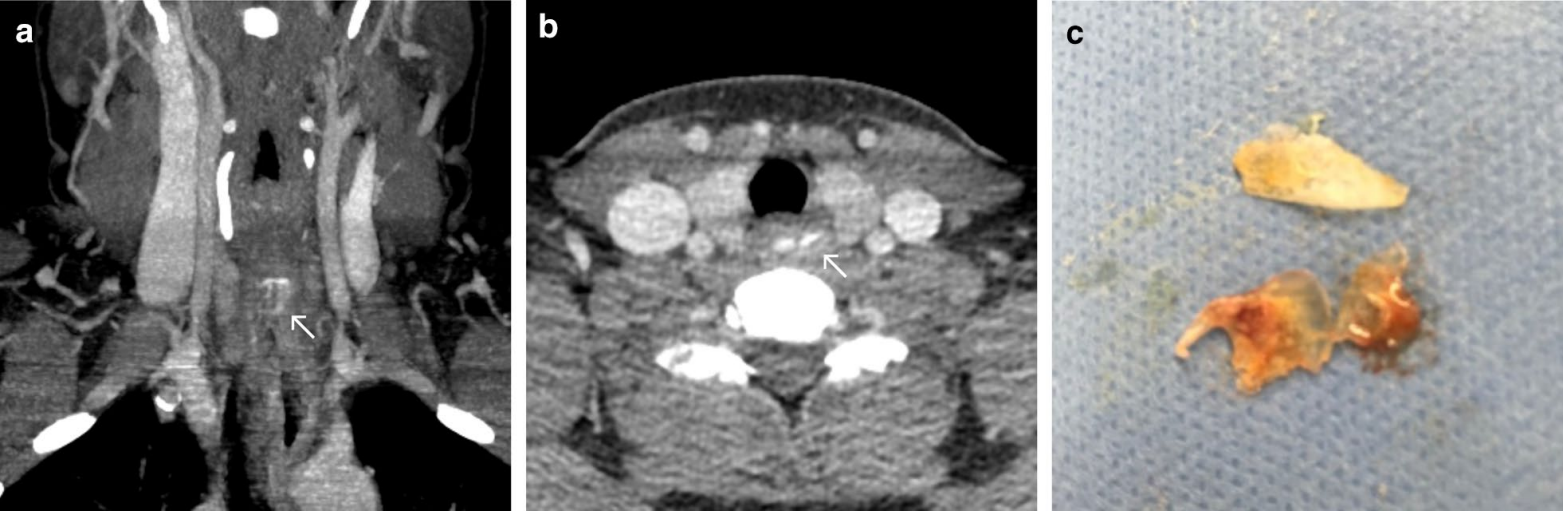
Fish scales. Coronal maximum intensity projection (a) and axial CT image (b) showing a flat hyperdense structure in the upper oesophageal sphincter. Photograph of multiple fish scales after endoscopic removal from the proximal oesophagus (c)
